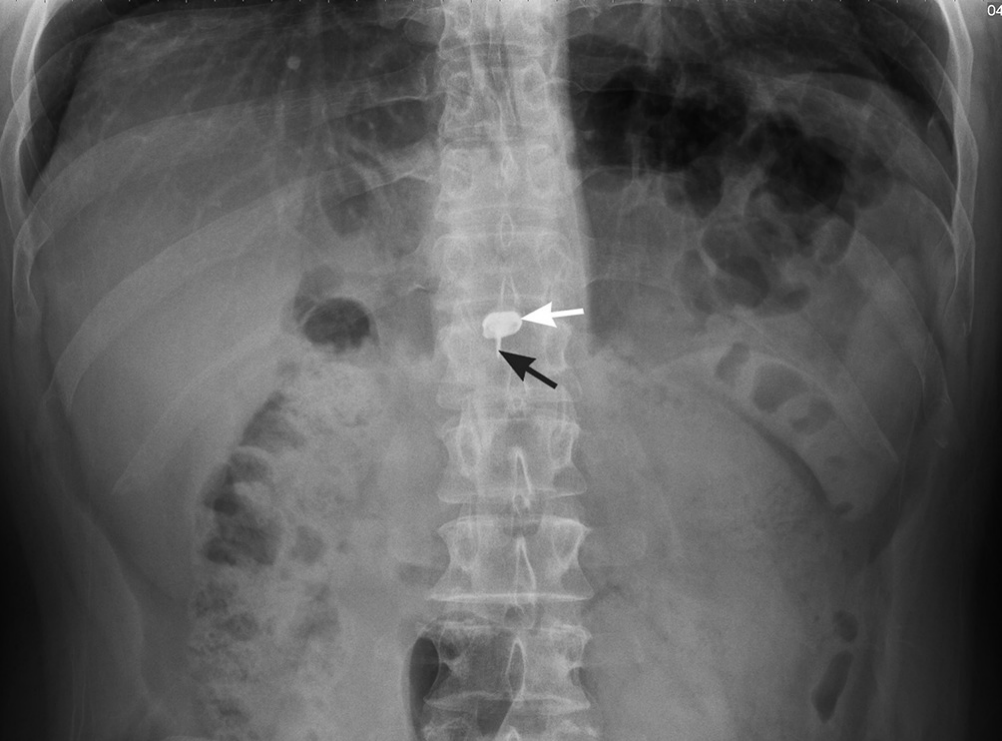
Supine abdominal anteroposterior radiograph shows a single crown (white arrow) with a post (black arrow) in the gastric antrum.
